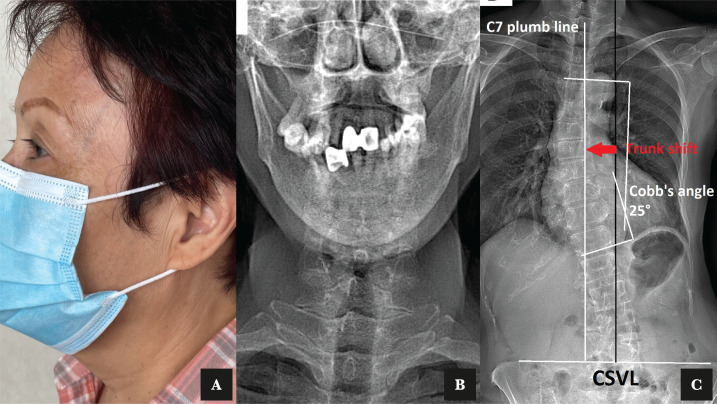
A – Repeat photography after treatment showed a normalization of the superficial temporal artery. B – Repeat radiograph after chiropractic care showed a normalization of the jaw. C – Repeat radiographs after treatments showed a reduction of lumbar scoliosis.
